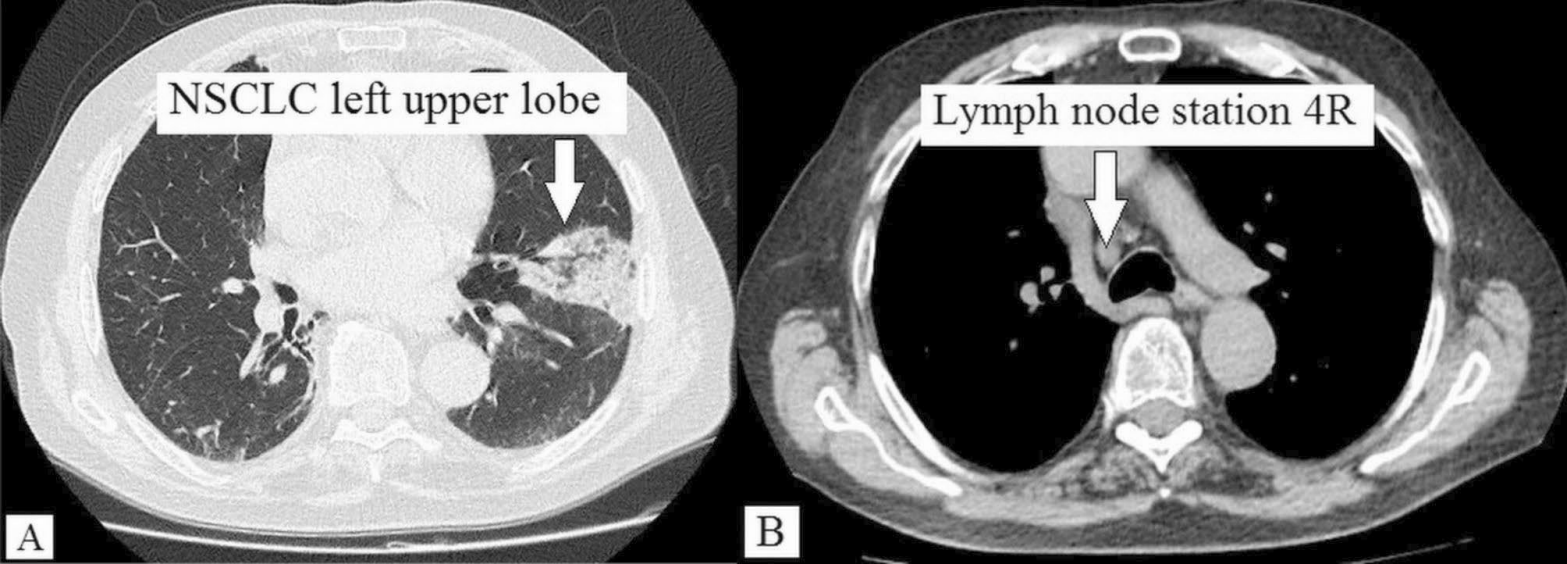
NSCLC of the left upper lobe (A). CT-enlarged mediastinal lymph node station 4R (B)
